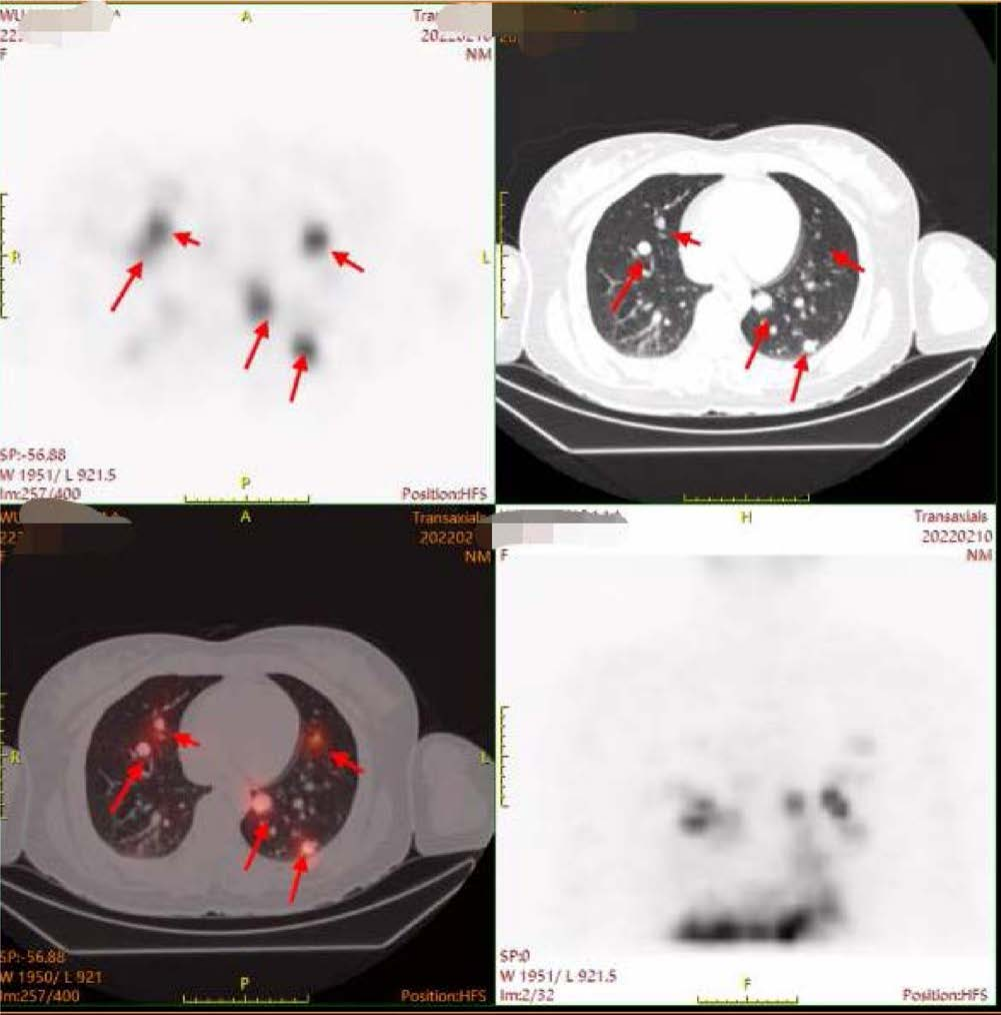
PET/CT of lung metastases in this patient. PET/CT = positron emission computed tomography.
